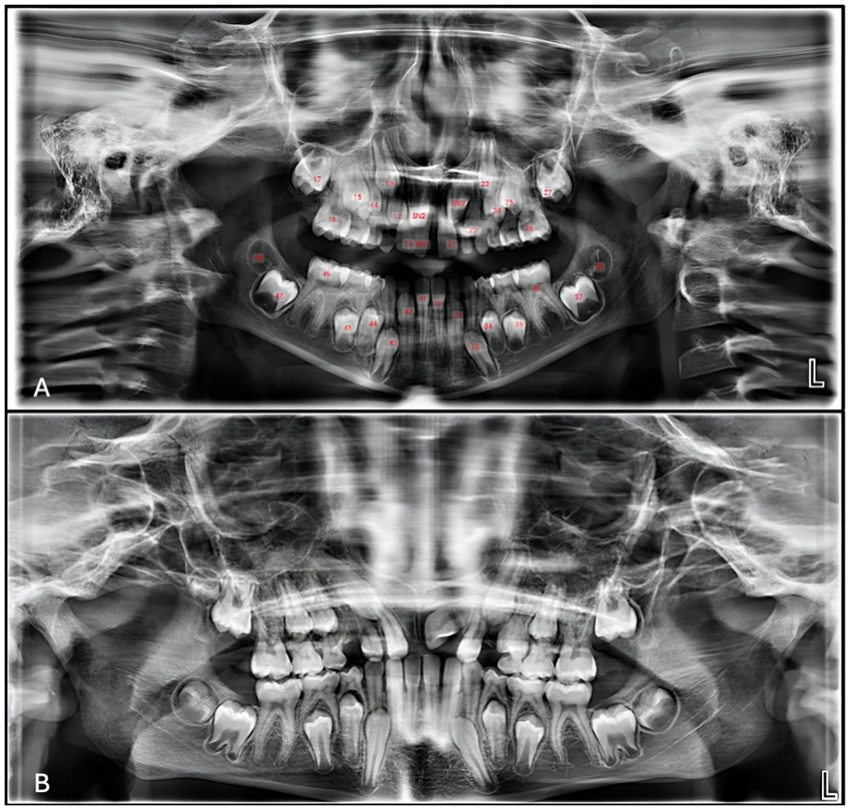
(A) Panoramic radiograph of tooth count shows multiple supernumeraries (SN) and right maxillary central incisor fused with SN1. (B) Panoramic radiograph revealing the redirection and eruption of SN2 and SN3.
